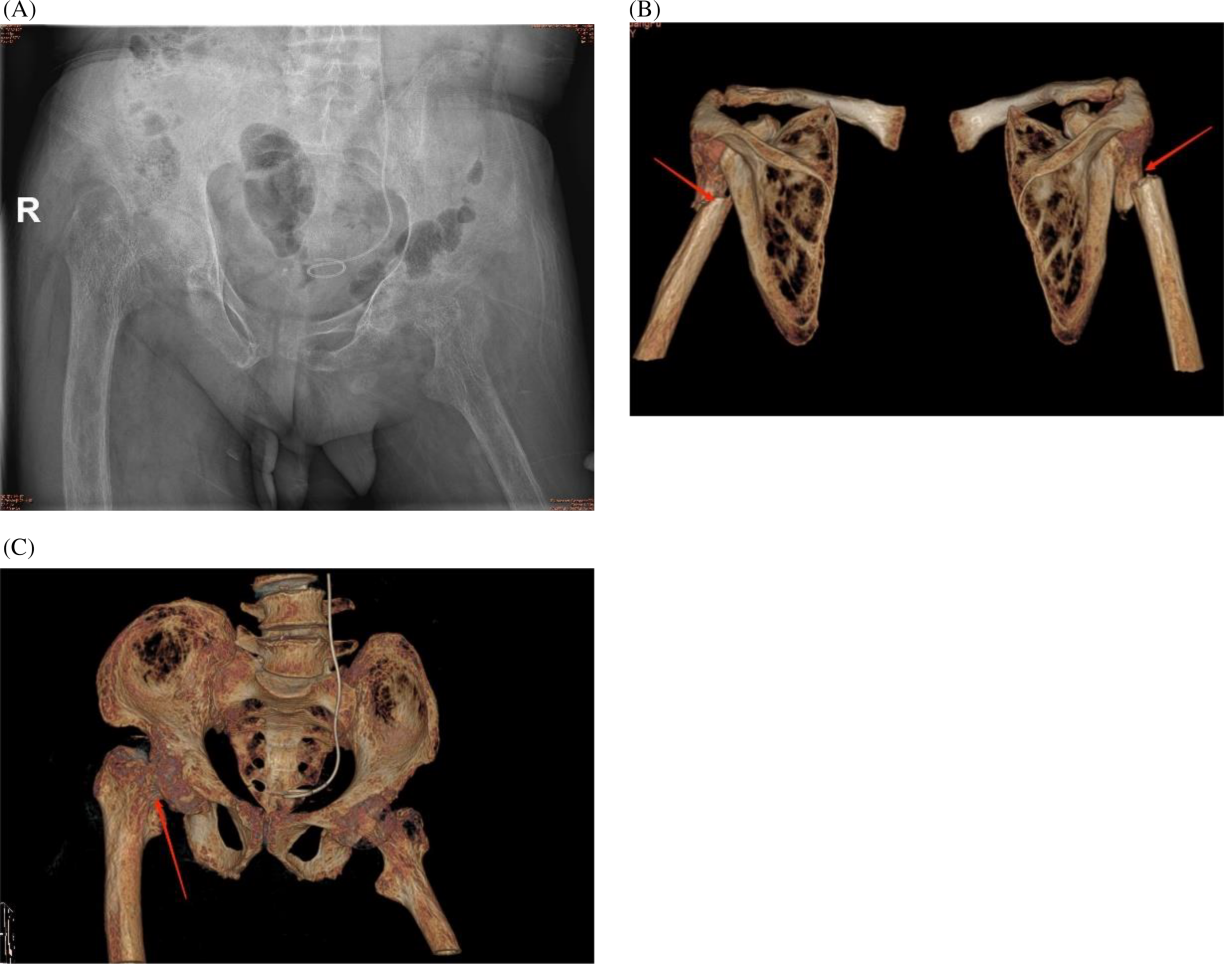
(A) X‐ray of the pelvis, showing a right femoral neck fracture and muscle atrophy around the left hip; (B) three‐dimensional computed tomography (CT) of the shoulder joints, presenting bilateral humeral shaft fractures; (C) three‐dimensional CT of the pelvis, presenting the fracture of the right femoral neck which alignment is good.
